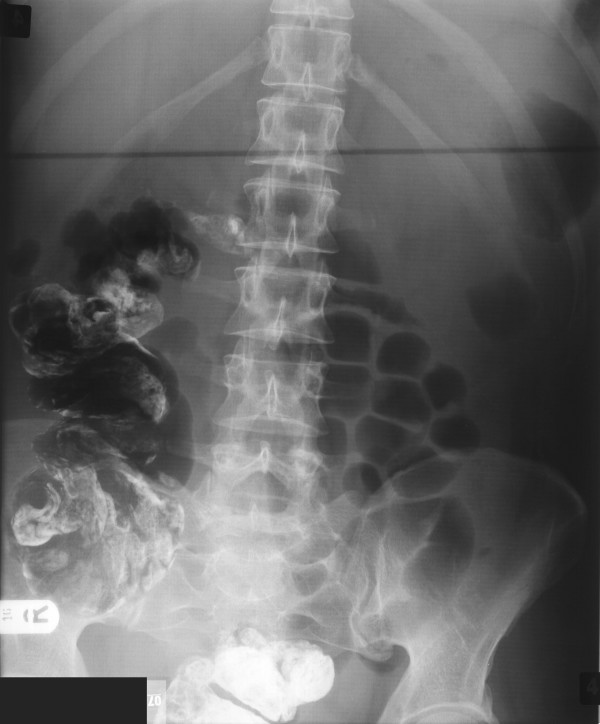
Abdominal radiograph demonstrating the large colon present in the abdominal cavity
